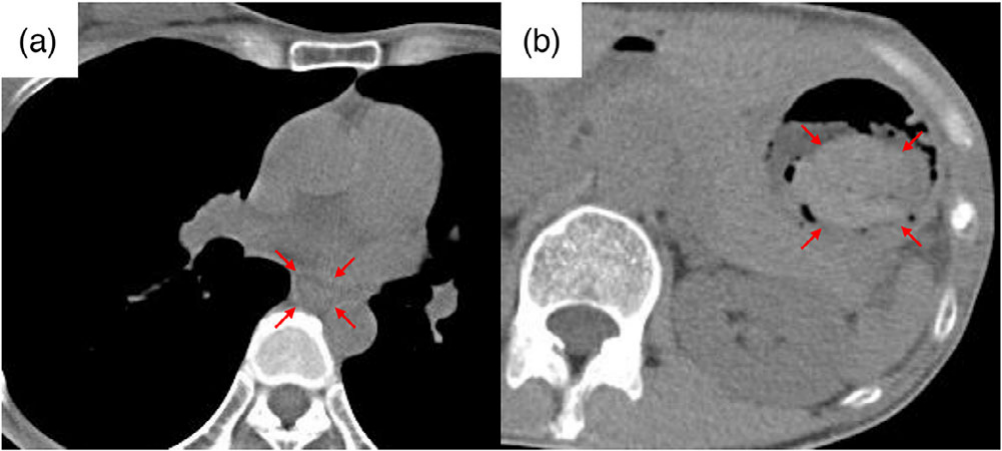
Plain computed tomography scan. (a) A continuous wall thickening from the middle to the lower thoracic esophagus was observed (red arrows). (b) A clearly demarcated hyperabsorptive zone, considered blood in the fundus of the stomach, was observed (red arrows).
